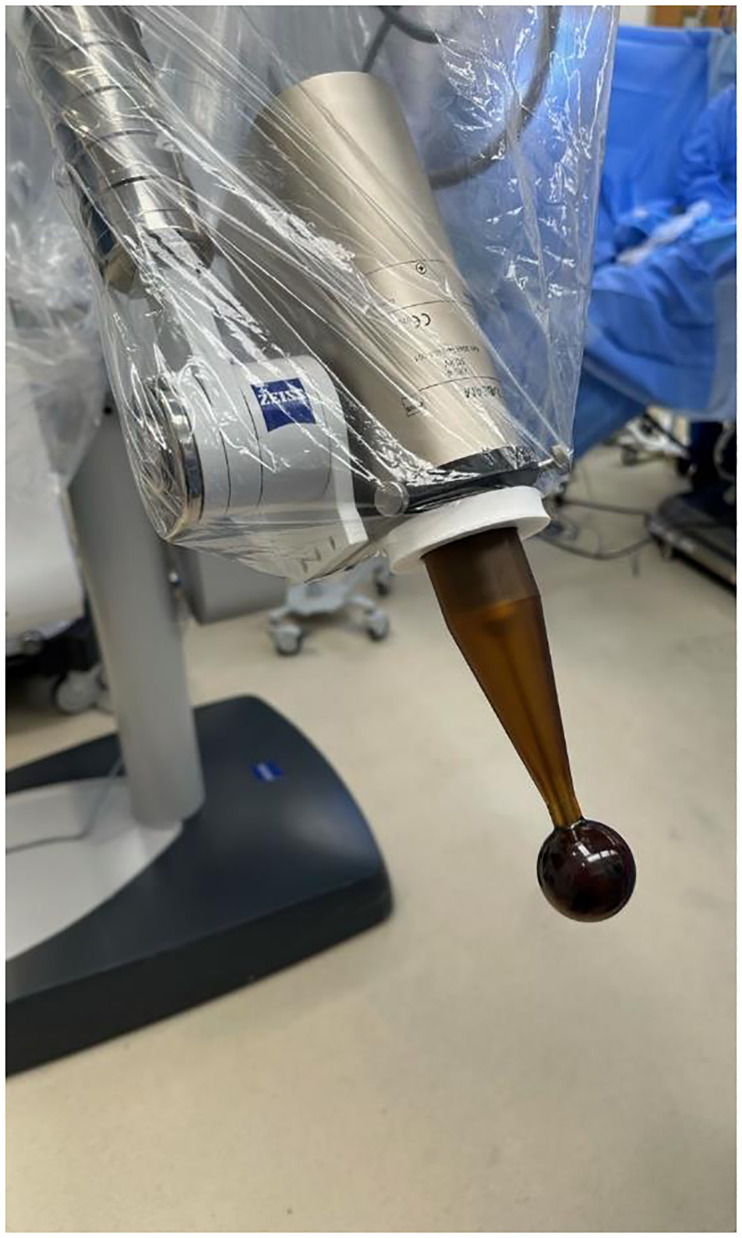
Dressed Intrabeam 600 miniaturized 50 KV X-Ray linear accelerator with 40 mm applicator mounted (Zeiss, Oberkochen, Germany).
